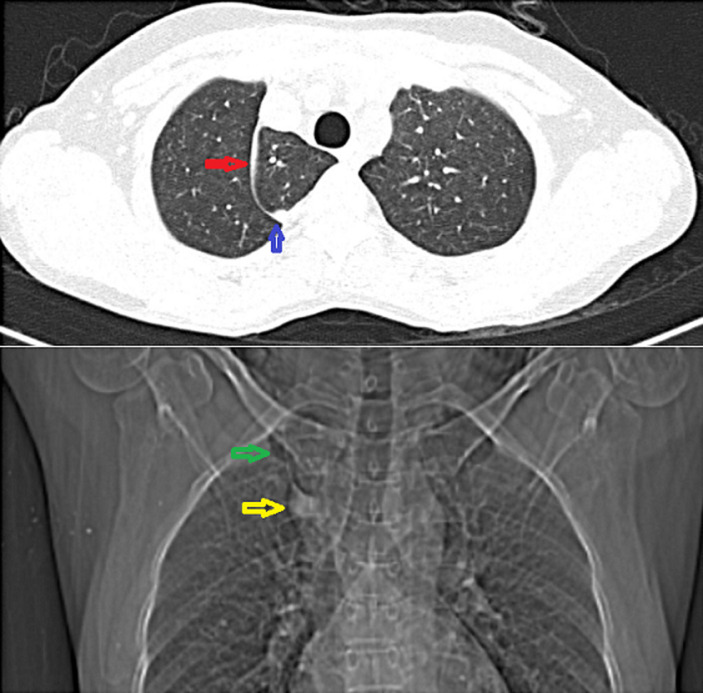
red arrow: an axial section of contrast-enhanced computed tomography of lungs showing azygos lobe separated from right upper lobe by azygos fissure; blue arrow: lower most part shows azygos vein; green arrow: zoomed in image of chest radiography showing azygos lobe along with azygos fissure; yellow arrow: azygos vein on right side
